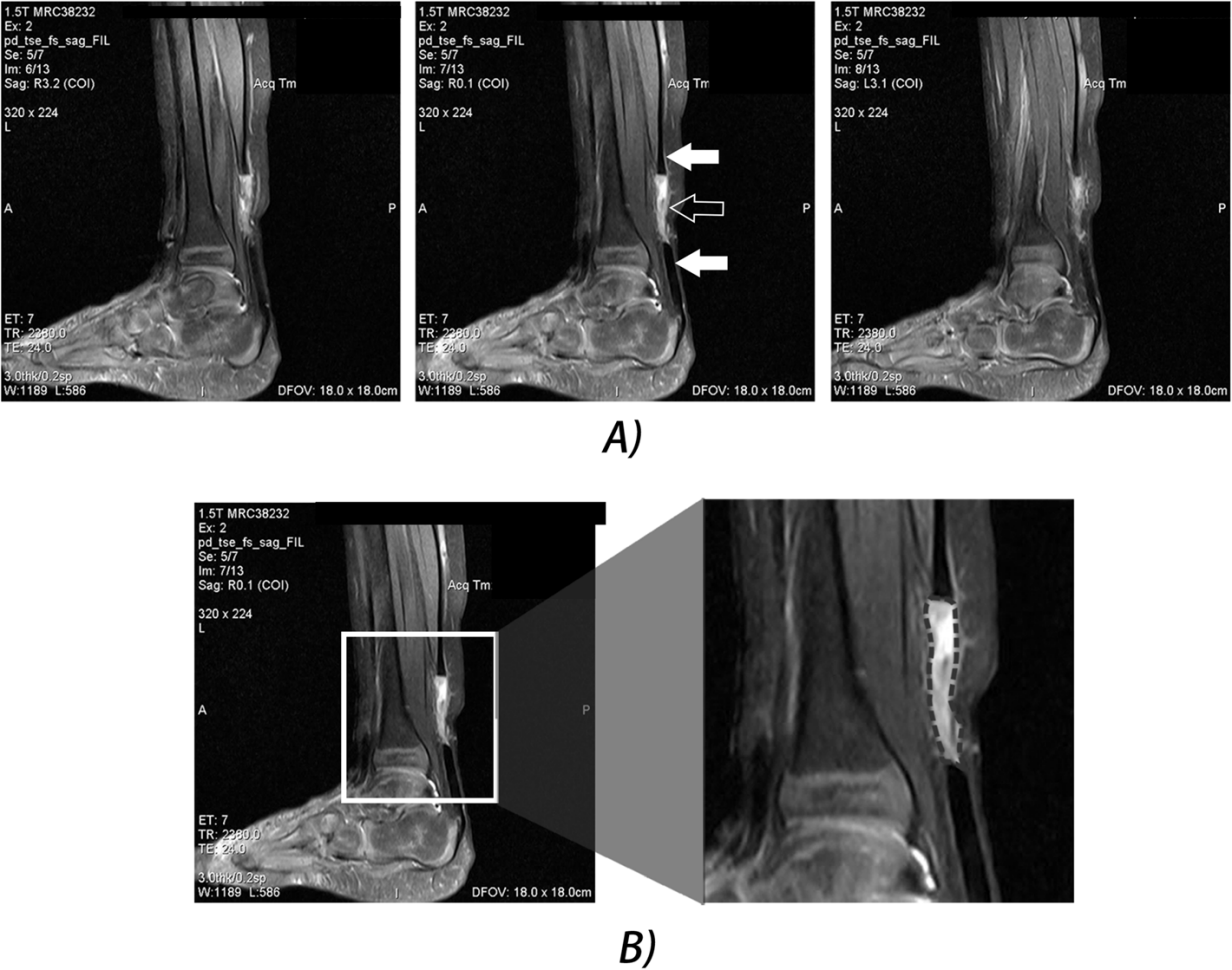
Example case of a sagittal PD-weighted MR image (TR/TE, 2380.0/24.0) where the area between the severed tendons was cropped. a From successive MR images, the middle image was chosen to represent the center of the tendon with respect to the sagittal cross-section. The middle image shows the severance of the tendon (white arrow) and the tendon gap (outlined arrow) with higher signal intensities than the surrounding muscle and tendons. b The area between the severed tendons was manually cropped and the pixel count of high signal intensity were computed in Python
